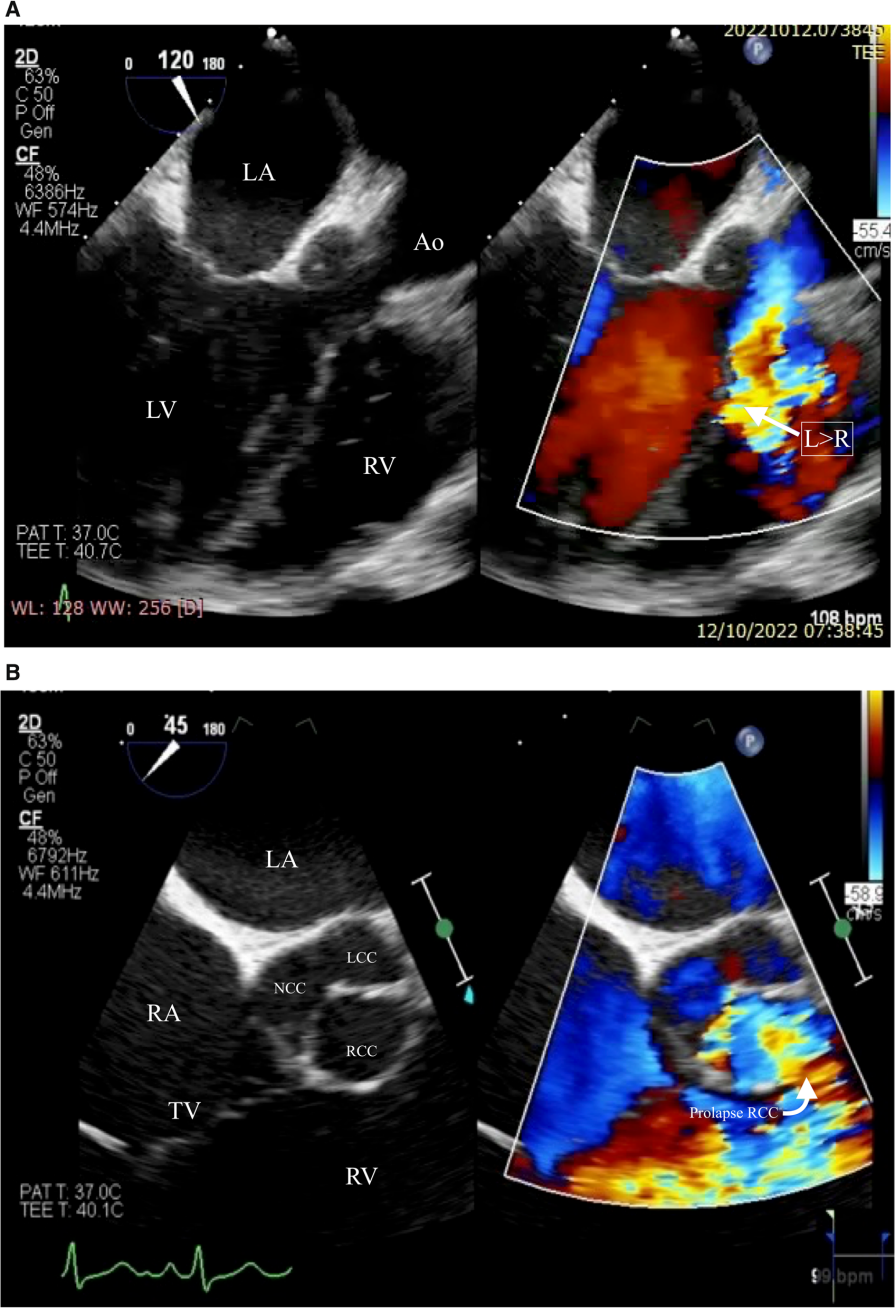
Transoesophageal echocardiography confirmed rupture of the sinus of Valsalva aneurysm on the Right coronary cusps (RCC). A and B Images taken flow from the RCC to the right ventricle on color doppler (arrow). Ao = aorta; RV = Right ventricle; LV = left ventricle; LA = left atrium; L = left; R = right; TV = tricuspid valve; NCC = non-coronary cusp; LCC = left coronary cusp; RCC = right coronary cusp
